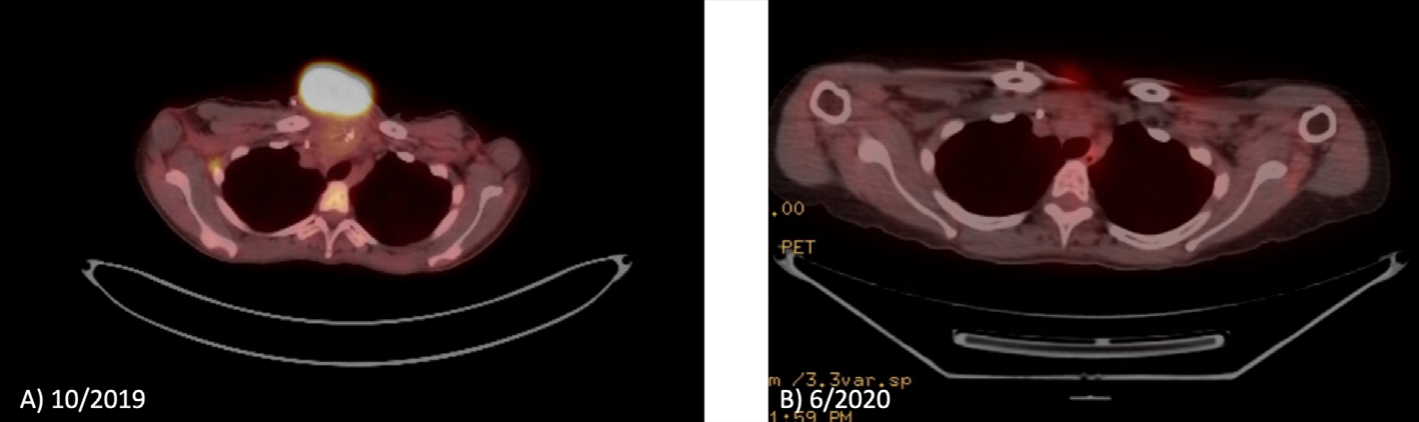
PET/CT before and after lenvatinib plus pembrolizumab on patient 1. (A) PET/CT demonstrates the chest well tumor before treatment. (B) PET/CT demonstrates near complete response of the chest well tumor.
